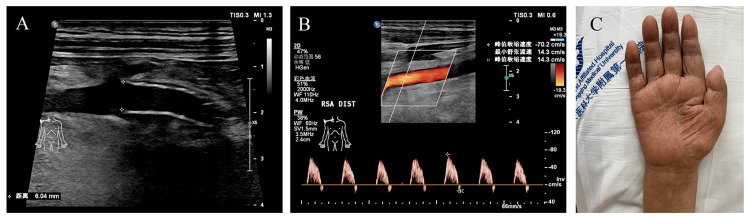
Six-month follow-up results. (A and B) Ultrasonography of the right subclavian artery demonstrates a well-formed intravascular stent (A) with unobstructed blood flow inside the stent (B). (C) Clinical manifestations of the right upper limb following endovascular treatment: erythematous skin color and complete healing of the ulcer on the right little finger.
